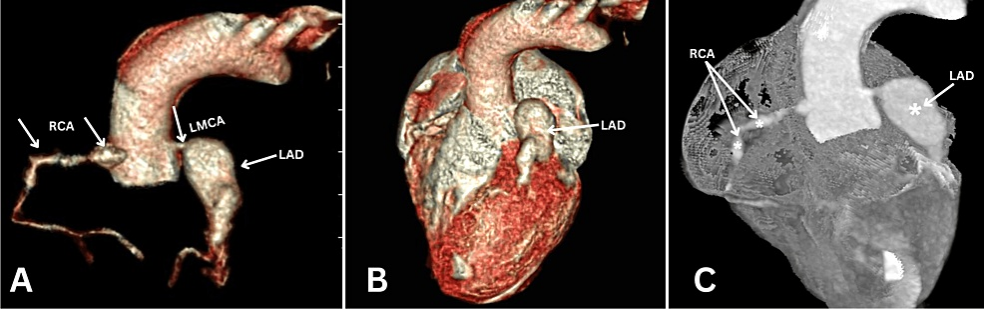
Comprehensive assessment of coronary artery involvement by CCTA: 3D VR image of (A) the aortic root with coronary vessels, (B) the whole heart, and (C) an outline MIP image of the heart, revealing several aneurysms in the proximal and mid-RCA (arrows and stars) and distal LMCA (arrows), and giant aneurysms in the proximal and mid-LAD (arrows and stars). CCTA: cardiac computed tomographic angiography; 3D: three dimensional; VR: volume rendered; MIP: maximum intensity projection; RCA: right coronary artery, LMCA: left main coronary artery; LAD: left anterior descending artery
